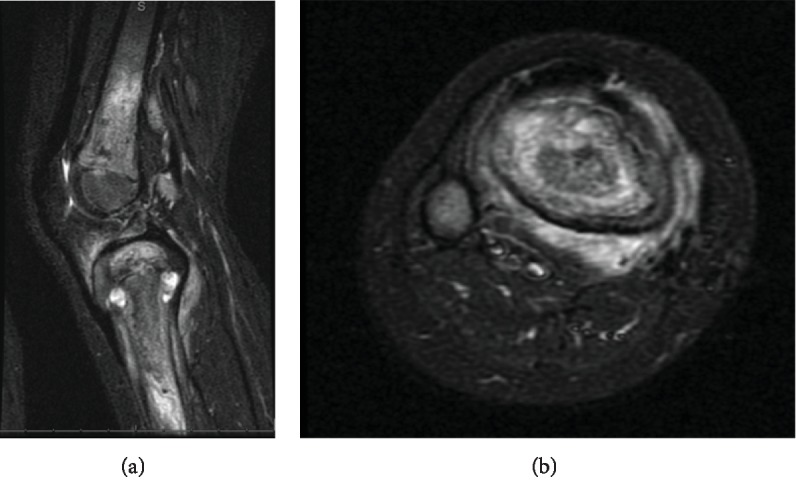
Sagittal (a) and axial (b) short tau inverted recovery (STIR) magnetic resonance imaging demonstrating extensive femoral and tibial edema consistent with osteomyelitis. In addition, two tibial abscesses distal to the physis and posteromedial muscle edema concerning for myositis were noted.
